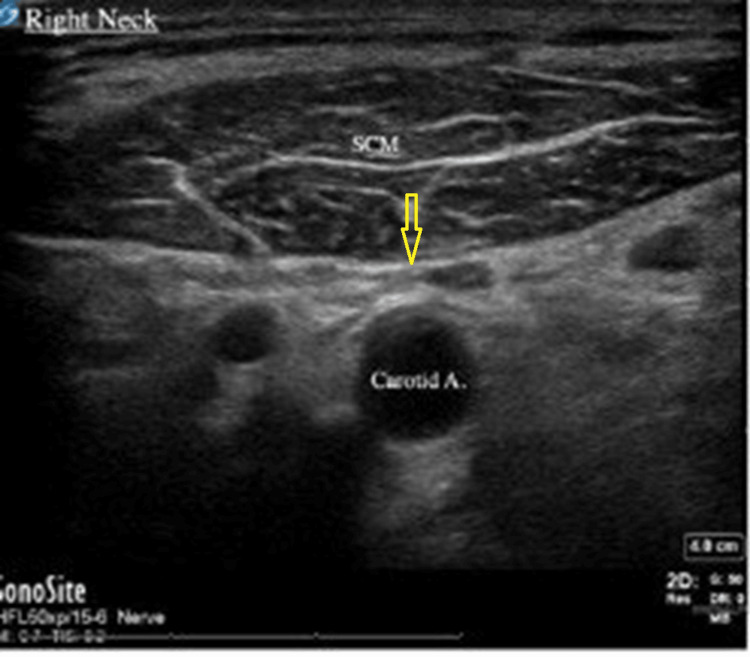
Ultrasound image of the right side of the neck showing yellow arrow at the expected site of internal jugular vein
